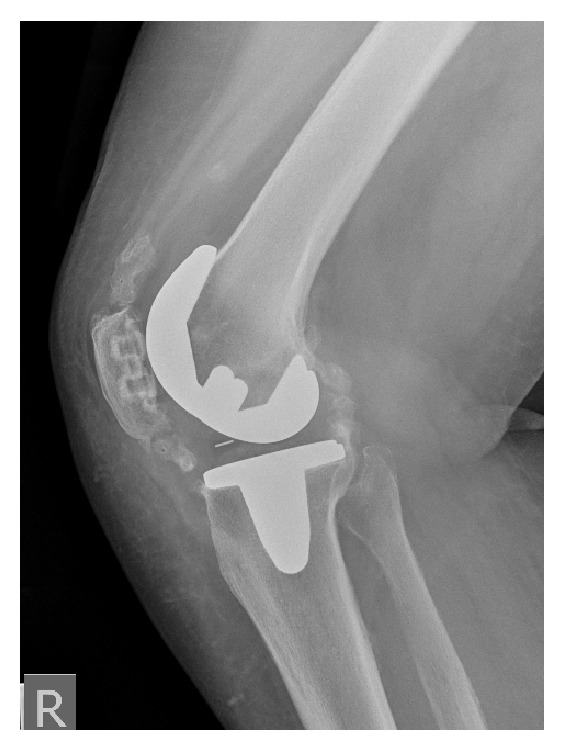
Postoperative radiographs of the total knee replacement at 8 months showing the severe heterotopic ossification.
